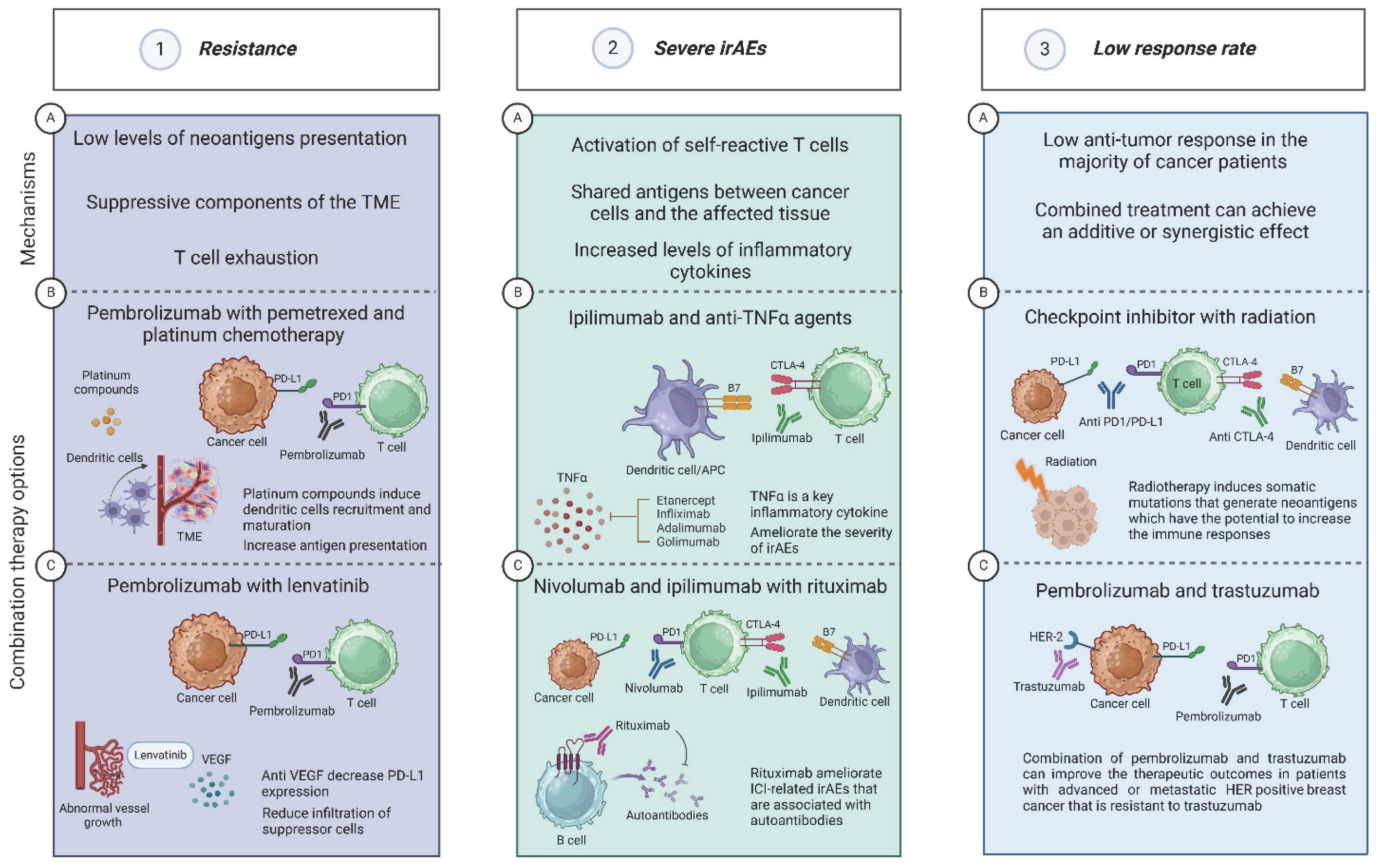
Different drug combinations with ICI can be used for different purposes. Panel 1. Resistance. 1A. The main mechanisms that can lead to the development of ICI-resistance are low levels of neoantigens presentation, suppressive components in the TME, and T cell exhaustion^35^,^38^,^41^. 1B. The combination of pembrolizumab with pemetrexed and platinum compounds can be used to overcome ICI-resistance. Platinum compounds induce dendritic cells recruitment and maturation and increase antigen presentation^43^-^45^. 1C. Pembrolizumab and anti VEGF such as Lenvatinib. Lenvatinib can decrease the levels of PD-L1 expression and reduce the infiltration of suppressor cells such as myeloid-derived suppressor cells (MDSCs) and regulatory T cells^54^,^55^. Panel 2. Severe irAEs. 2A. The main mechanisms that can lead to the development of irAEs are activation of self-reactive T cells, shared antigens between cancer cells, and increased production of inflammatory cytokines and chemokines^69^-^71^. 2B. The combination of ipilimumab and anti-TNFα agent such as infliximab was shown to be effective for treating corticosteroid-refractory ICI-related colitis^78^. In addition, adalimumab was successful in ameliorating the severity of ICI-related joints inflammation^79^. 2C. AntI-CD20 agents such as rituximab were effective in ameliorating ICI-related irAEs that are associated with the detection of autoantibodies such as encephalitis^89^. Panel 3. Low response rate. 3A. There is an ongoing effort to find new strategies to enhance the anti-tumor response since most cancer patients will not achieve the treatment goals^17^. One of these strategies is to gain an additive or synergistic effect by using combination therapy. 3B. Combining ICIs with radiotherapy has the potential to improve the treatment outcome. Following radiotherapy, some patients may express somatic mutations that generate neoantigens which have the potential to increase the immune responses^97^,^98^. 3C. Another significant motivation to explore new CT with ICIs is to increase the range of indications for additional types of cancer, such TNBC. The combination of trastuzumab (an anti-HER-2 mAb) with pembrolizumab has been evaluated in clinical trials in patients with advanced or metastatic HER positive breast cancer that is resistant to trastuzumab. The data gathered from this trial showed promising therapeutic outcomes including 15% response rates in PD-L1-positive tumors with 13% 12-month PFS^111^. Created with BioRender.com.
